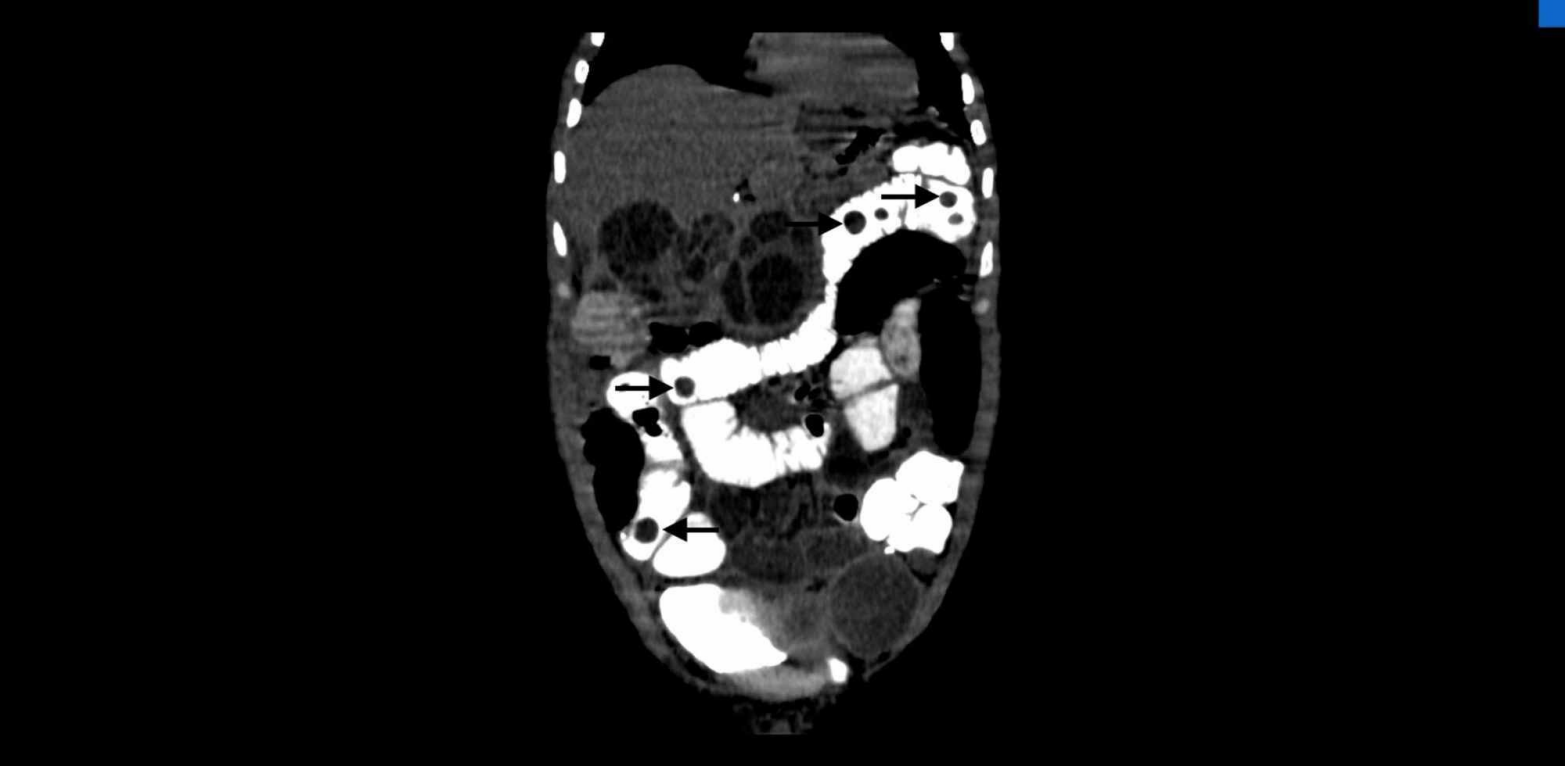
Coronal CT image shows multiple intraluminal cysts within the small bowel. Black arrows depict intraluminal small bowel cysts.
